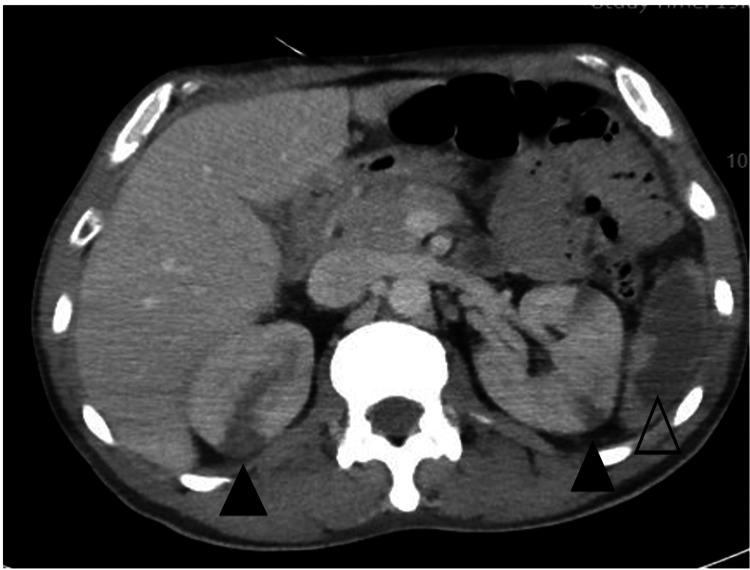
Bilateral renal infarcts (black arrowhead) and extensive splenic infarct (transparent arrowhead) on CT abdomen.
